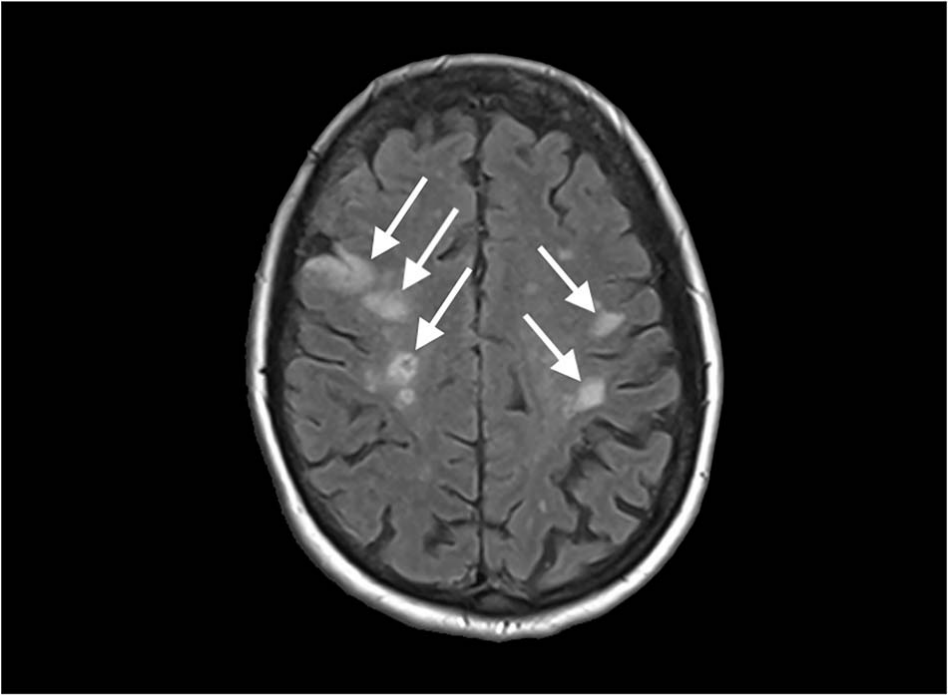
Diffusion-weighted magnetic resonance imaging image of the brain, showing multiple lesions.
